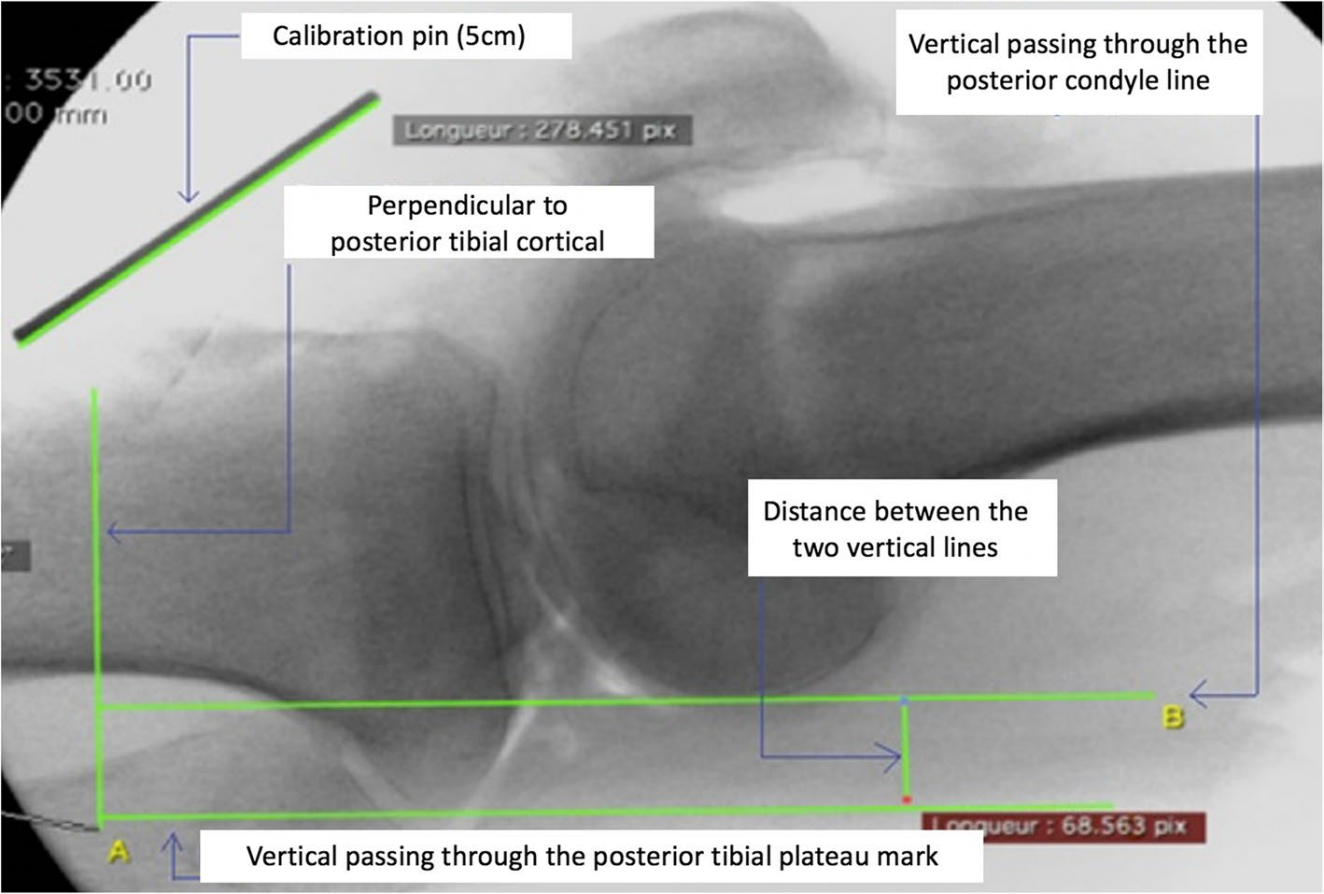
Analysis of a lateral radiographic image of the knee in extension with posterior stress with the conventional translation technique measurement
